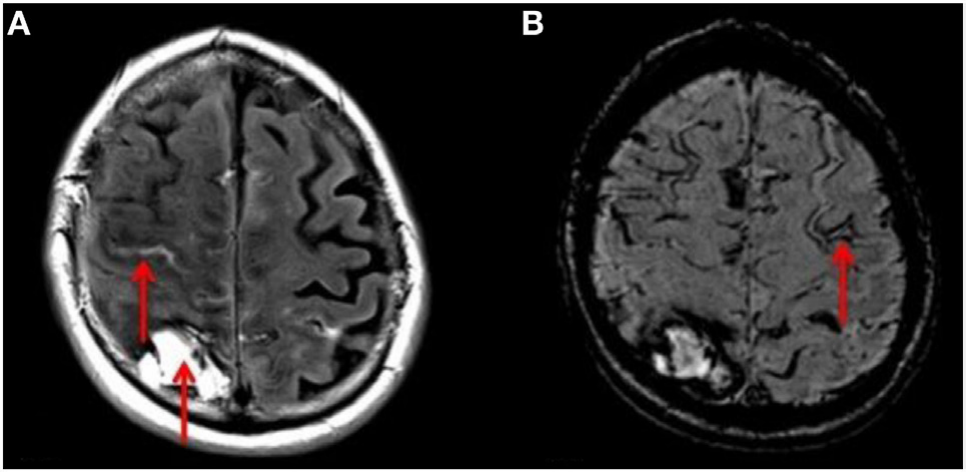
(A) T2 FLAIR axial MRI of the brain showing right-sided ICH and acute bleeding (red arrows). (B) SWI sequence MRI of the same patient demonstrating a linear gyriform hypointensity in a cortical distribution (red arrow), representative of siderosis, in a region independent to that of the site of hemorrhage.
